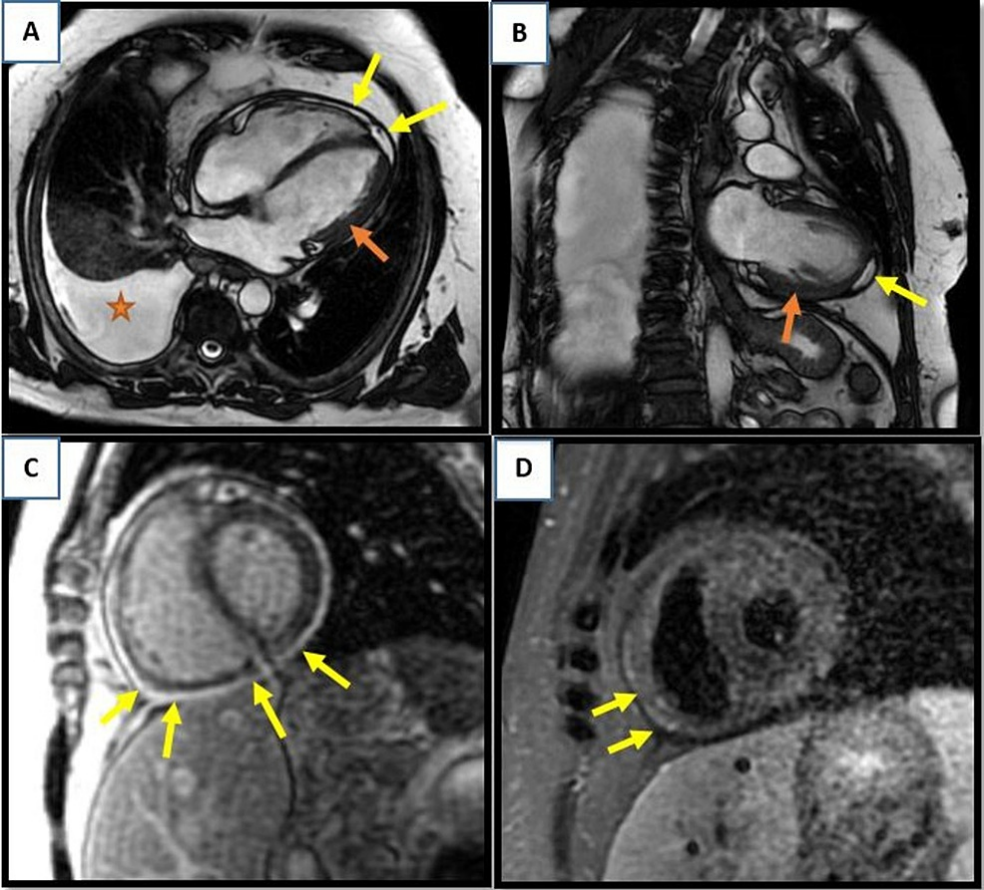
Cardiac MRI. (A) Cross-sectional four-chamber and (B) coronal steady-state free precession (SSFP) images showing thickened pericardium (red arrows), small pericardial effusion (yellow arrows), and right-sided pleural effusion (red star). (C) Diffuse pericardial delayed enhancement is consistent with pericardial inflammation (arrows). (D) T2-weighted image showing thickened pericardium (arrows).
